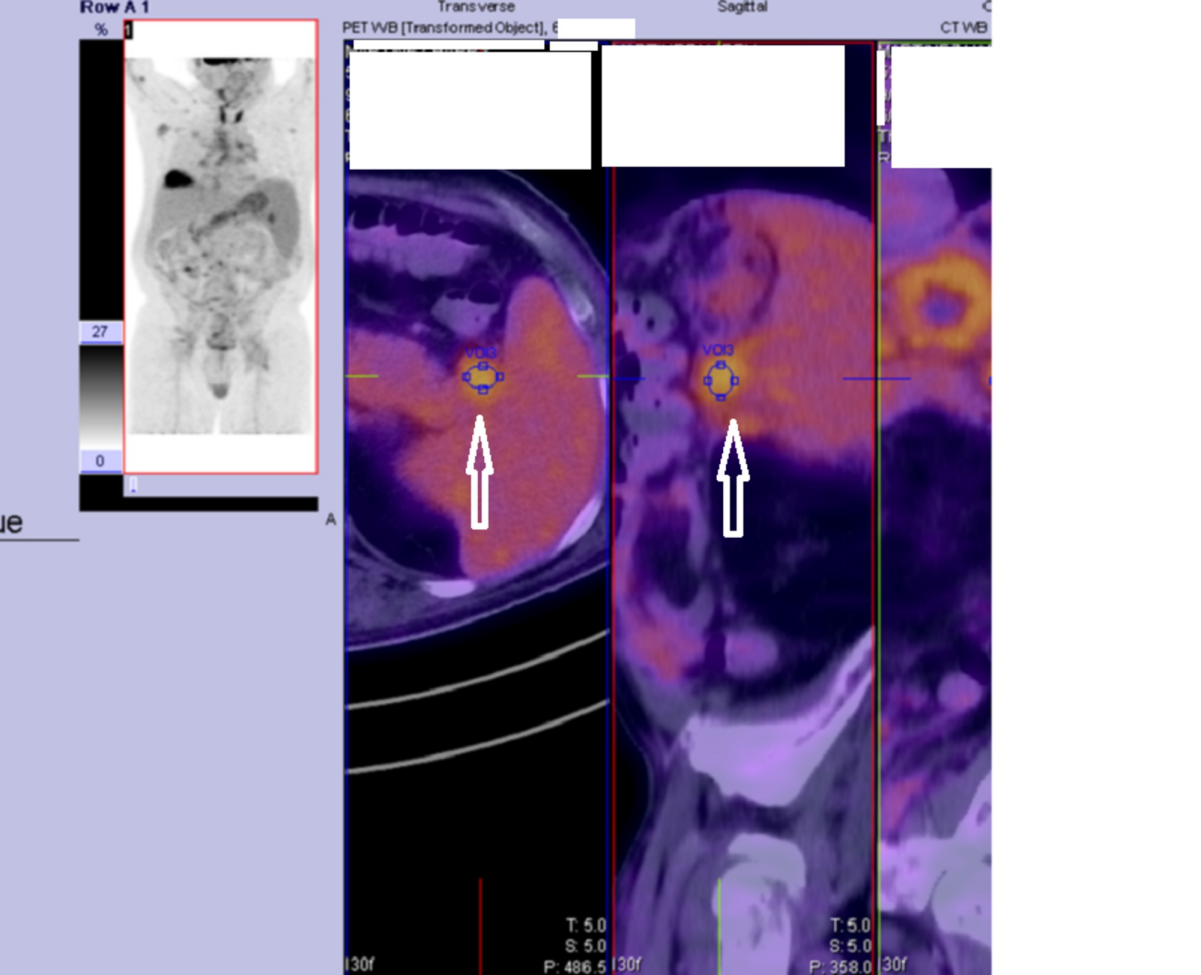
Abdominal PET-CT. PET-CT, positron emission tomography-computer tomography. Arrows:  enlarged lymph nodes with increased metabolic activity adjacent to an enlarged spleen, which also exhibits increased metabolic activity.
